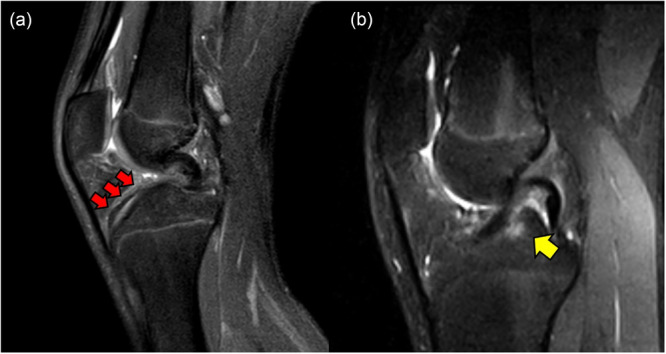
Presence of a hyperintense signal band between the fibres alongside a low‐intensity signal within the graft (a) and a hyperintense signal area in the cancellous bone around the graft (b) at short tau inversion recovery (STIR) magnetic resonance imaging scans.
